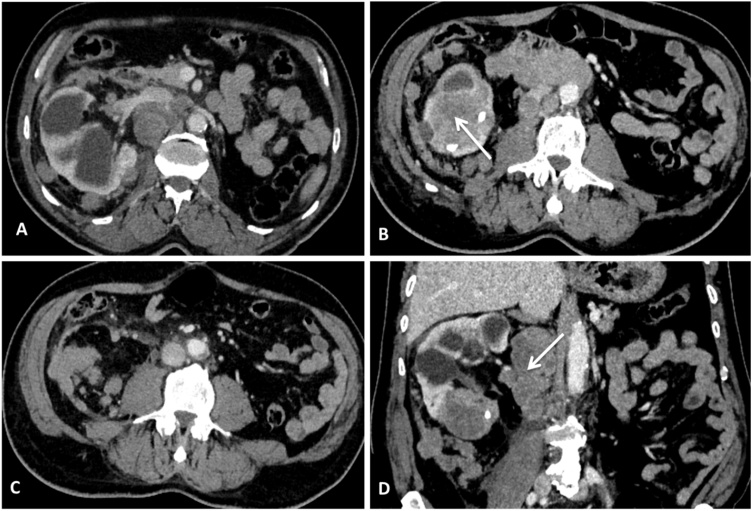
CT scan showing (A): hydronephrotic solitary right kidney contiguous nodules in the perirenal fat (B): a heterogeneous mass in the lower pole of the kidney (arrow) with renal calculi (C) multiple nodules in the perirenal fat (D): a coronal plane showing the mass and the voluminous lymph node compressing the inferior vena cava (arrow).
